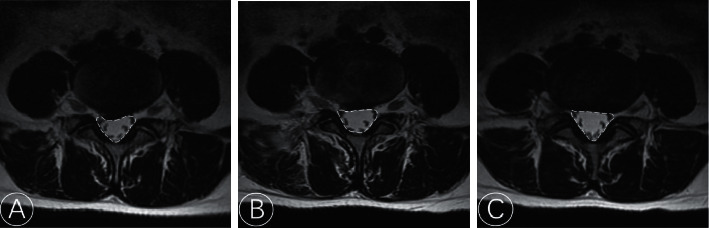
Comparison of spinal cross-sectional area (SCSA) on MRI preoperatively (a), at 3 days (b), and at 1 year after operation (c).
